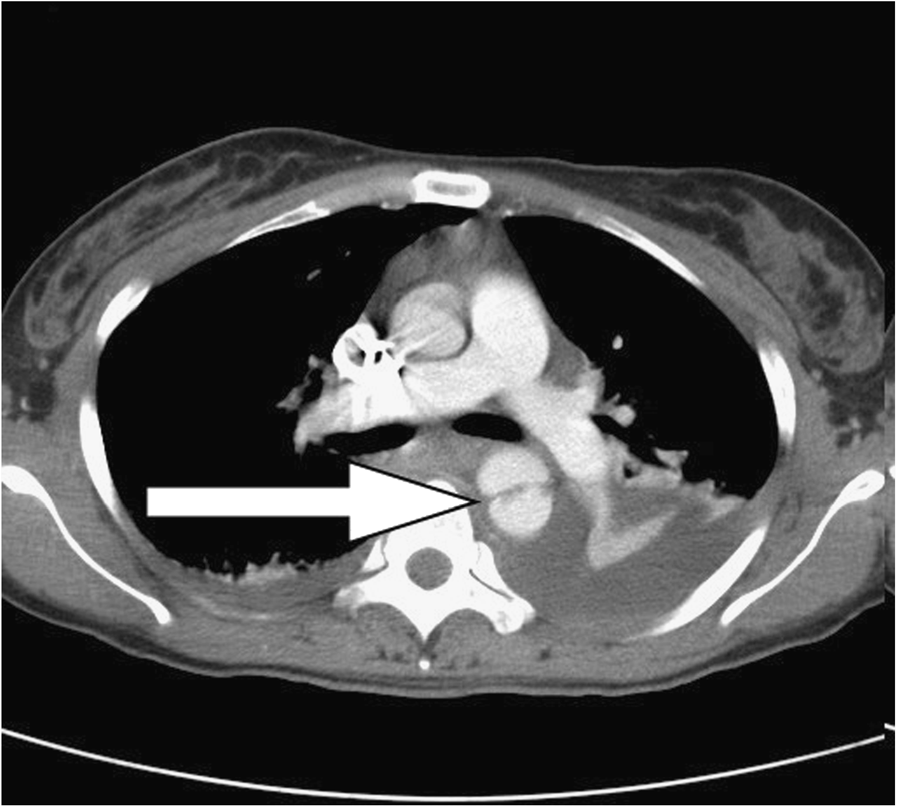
The axial view of computed tomographic angiography (CTA) revealed a dumbbell-shaped aorta (arrow) with hemothorax and hemomediastinum consistent with a ruptured thoracic aortic pseudoaneurysm.
